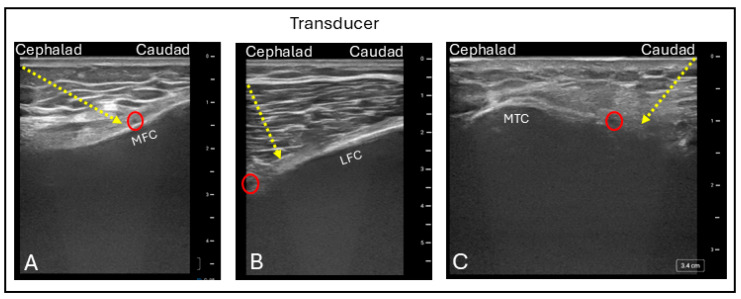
Ultrasound images demonstrating: (A) The in-plane superomedial genicular nerve block technique; (B) the superolateral genicular nerve block technique; and (C) the inferomedial genicular nerve block technique. Red circle = Genicular artery; MFC = Medial femoral condyle; LFC = Lateral femoral condyle; MTC = Medial tibial condyle; Dashed line = needle trajectory.
